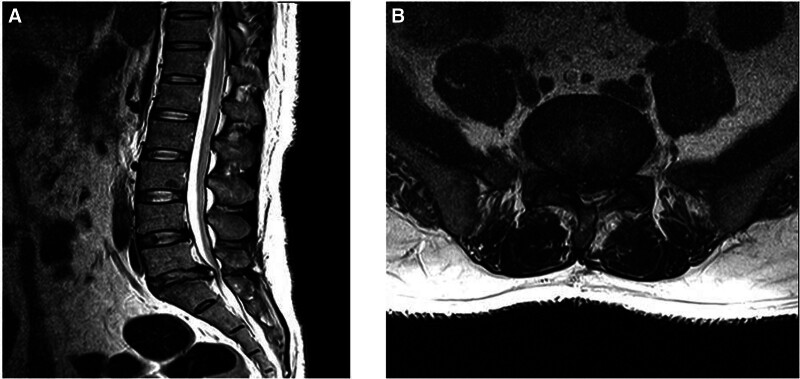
(January 14, 2022) L-spine magnetic resonance imaging: postoperatively. (A) T2 sagittal view; (B) T2 axial view.
